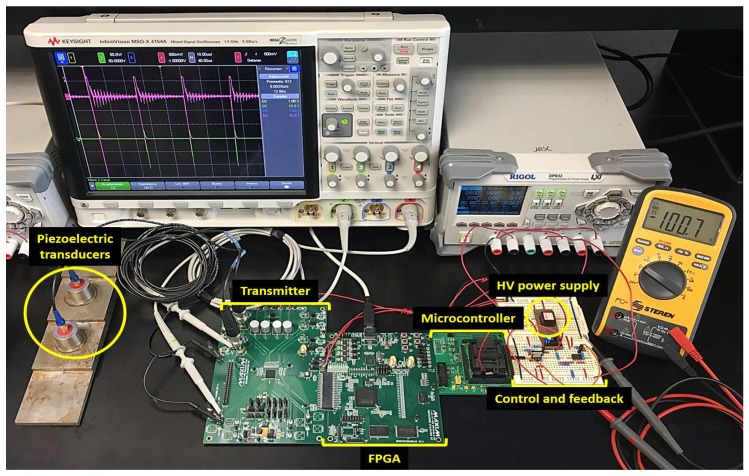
Ultrasound system used for experimental testing.
